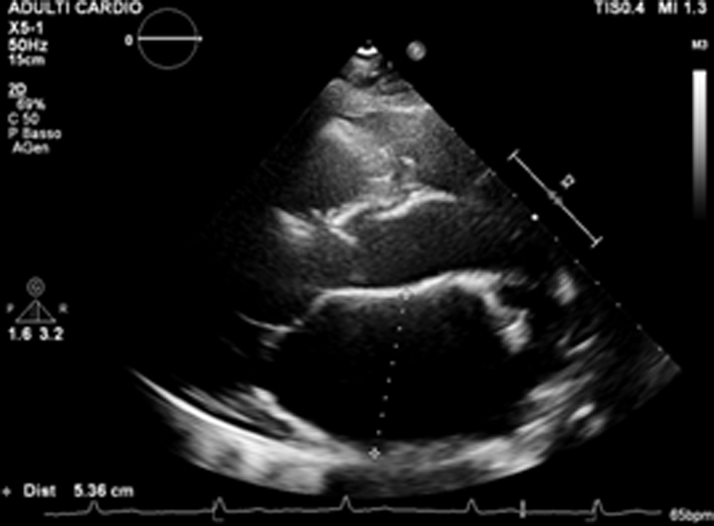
Parasternal Long-Axis Transthoracic Echocardiographic View of the Proband Showing a Markedly Enlarged Left Atrium, With an Anteroposterior Diameter Measuring 54 mm
